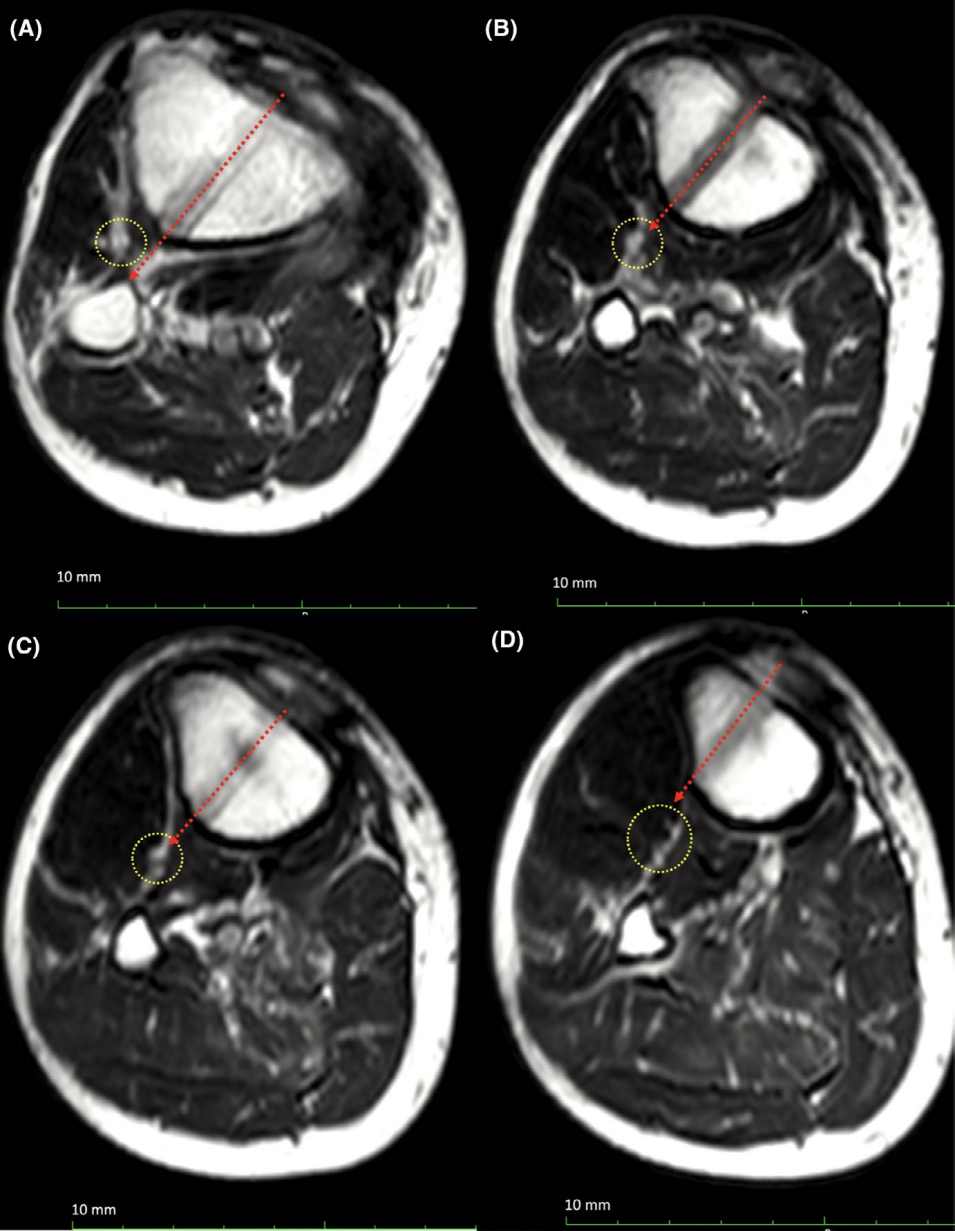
Magnetic resonance imaging at the level of distal locking screw holes (A, #1; B, #2; C, #3; D, #4) indicating the location of the neurovascular bundle including the deep peroneal nerve (yellow dotted circle) and direction of drilling for screw hole (red dotted arrow)
